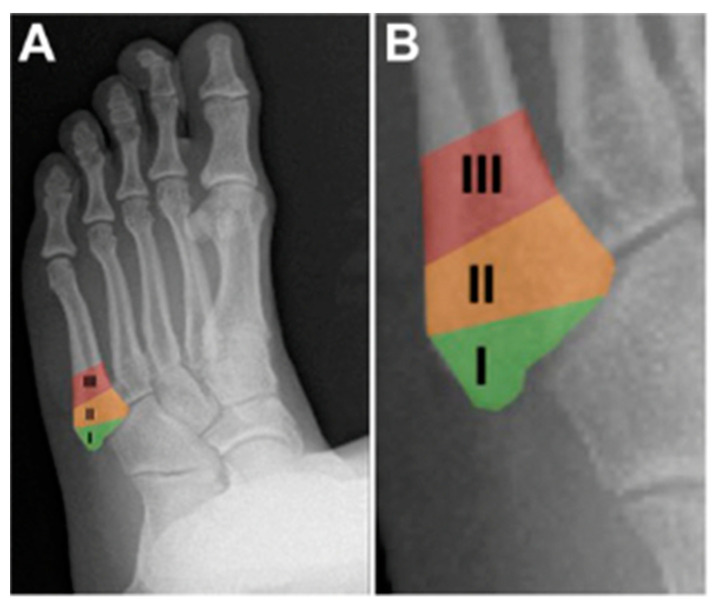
Normal radiograph of a left foot. (A) The 3 zones: Zone 1 (tuberosity avulsion fracture), Zone 2 (Jones fracture), and Zone 3 (proximal diaphyseal fracture (stress fracture)). (B) Closer view of the zones [5].
